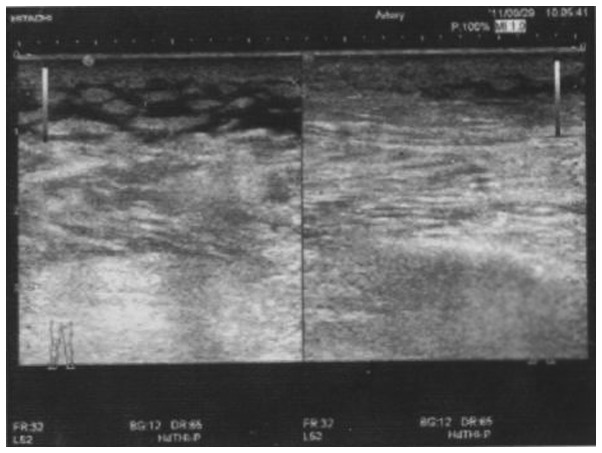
Ultrasonography revealed subcutaneous edema of the lower limbs, which was more marked in the left limb. No gas formation was observed in either leg and deep vein thrombosis was also not observed.
